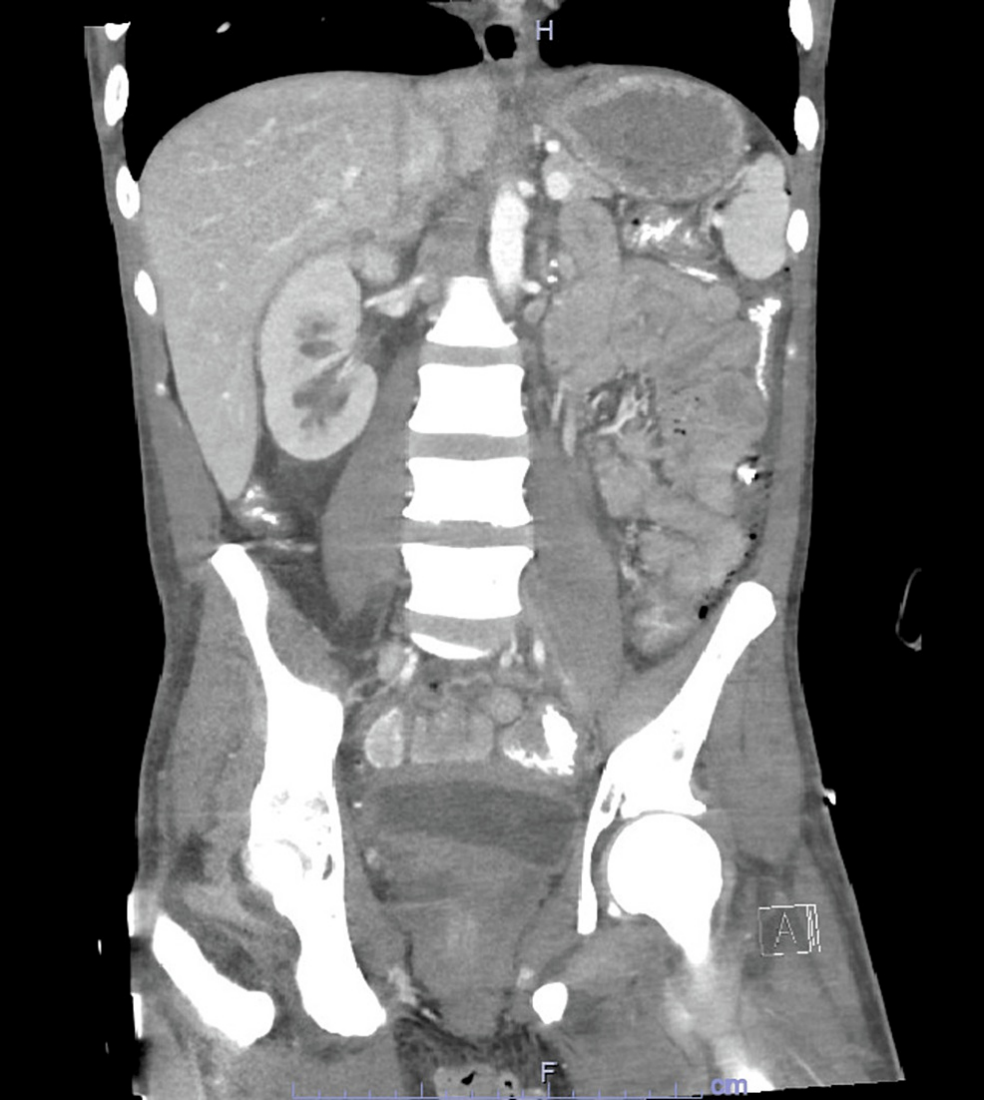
Post Nephrectomy CT Scan Post left-sided nephrectomy CT scan of the abdomen coronal section.
